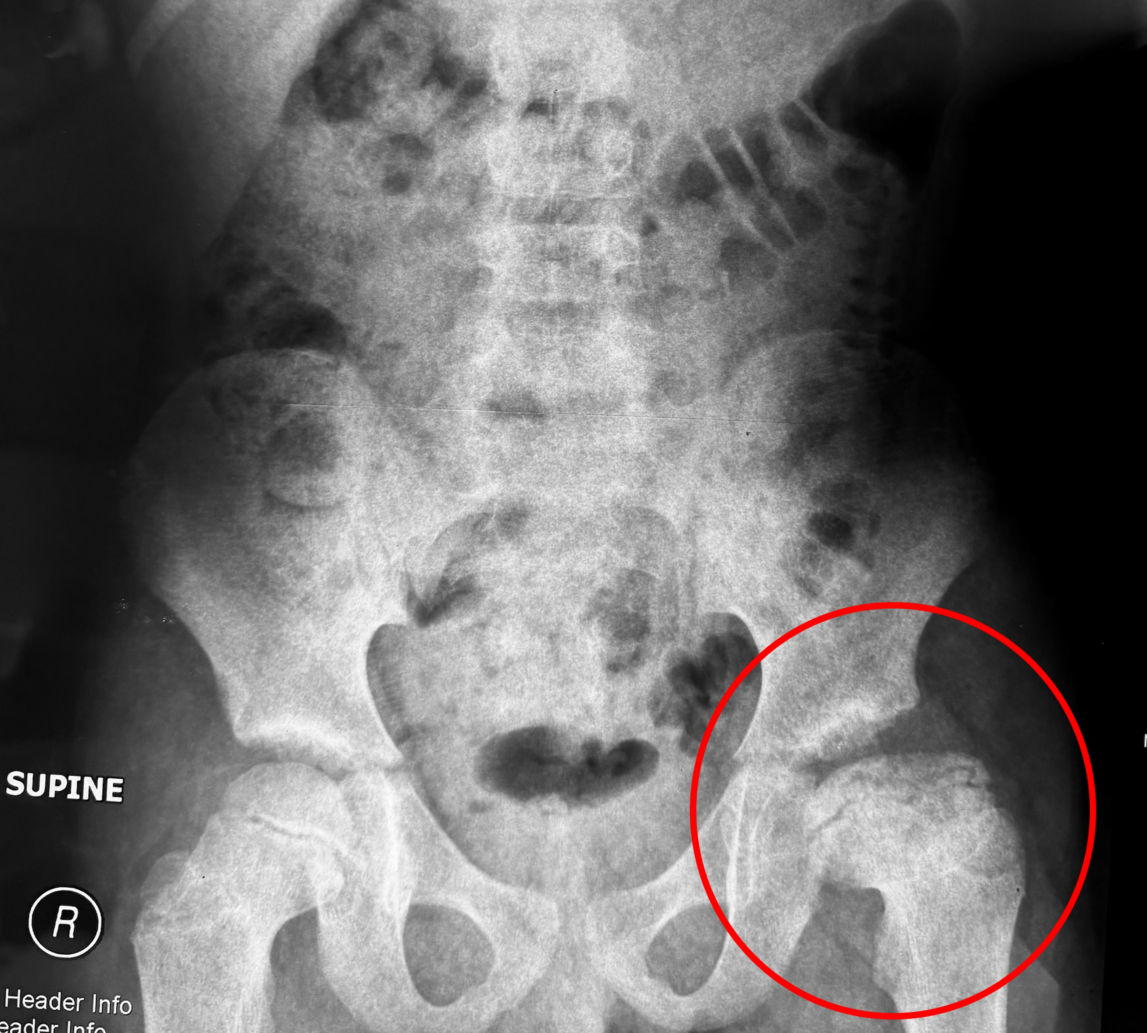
Supine anteroposterior pelvic X-ray taken on December 28, 2009.
